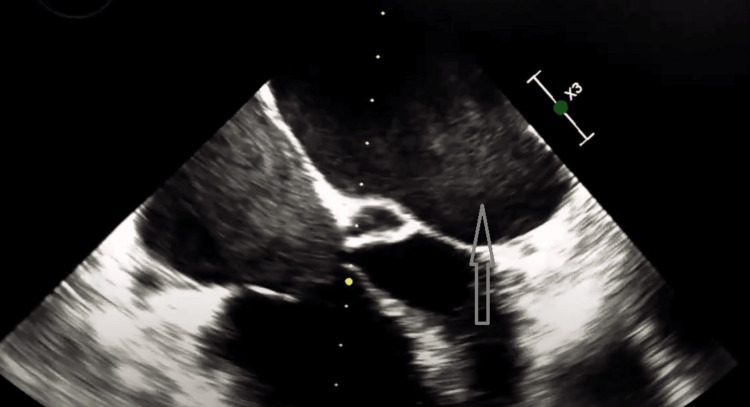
Transthoracic echocardiography Gray arrow showing dense spontaneous echocardiographic contrast in the left atrium.
